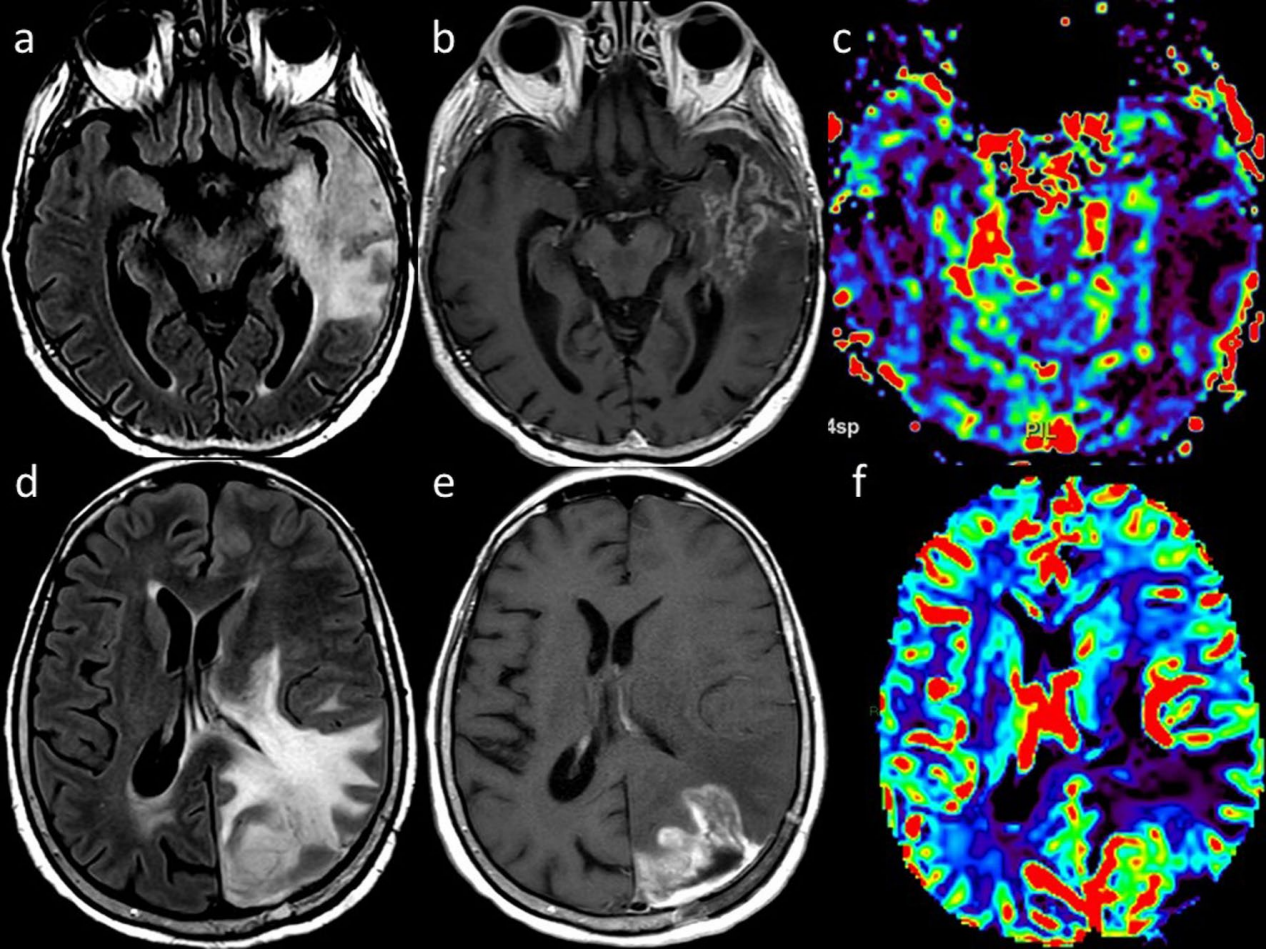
Radionecrosis versus recurrent tumor. Axial FLAIR (a), contrast-enhanced T1-weighted (b) and DSC rCBV map (c) of a 72 year-old man with GBM treated with surgery and ChT/RT show brain swelling, high FLAIR signal, low”soap bubble” enhancement and reduced rCBV in the left temporal lobe, consistent with radionecrosis. Axial FLAIR (d), contrast-enhanced T1-weighted (e) and DSC rCBV map (f) of a 52-year-old woman with glioblastoma treated with surgery and ChT/RT show brain swelling, high FLAIR signal, intense enhancement and increased rCBV, indicative of tumor recurrenceTransient focal enhancing lesions have been recently described with focal T2-hyperintense lesions and “snowflakes” or curvilinear enhancement in the pons, cerebellum, and posterior cerebral hemispheres [14]. Lastly, it must be pointed out that irradiated patients have sevenfold increased risk of developing secondary brain tumors, specifically meningioma, sarcoma and malignant glioma [11, 14].Stroke-like migraine attacks after RT:It is an uncommon delayed complication [14]. MRI shows unilateral temporal, parietal, or occipital gyriform T2/FLAIR hyperintensities and enhancement that do not respect vascular territories, while DWI may reveal superimposed infarcts [14].
